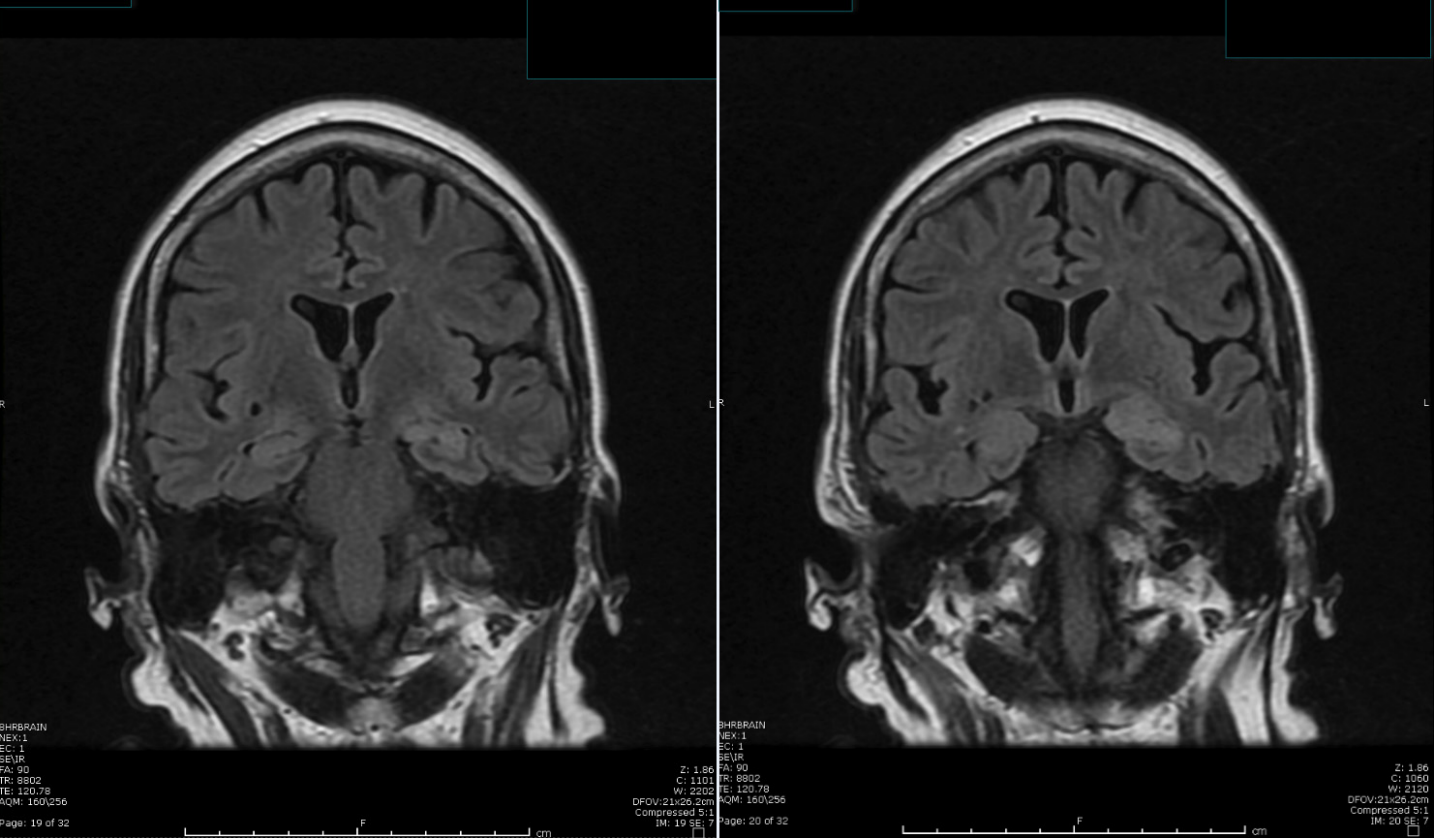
MRI of the brain showing subtle increased signal intensity on coronal FLAIR MRI sequences in both hippocampus (left > right).
